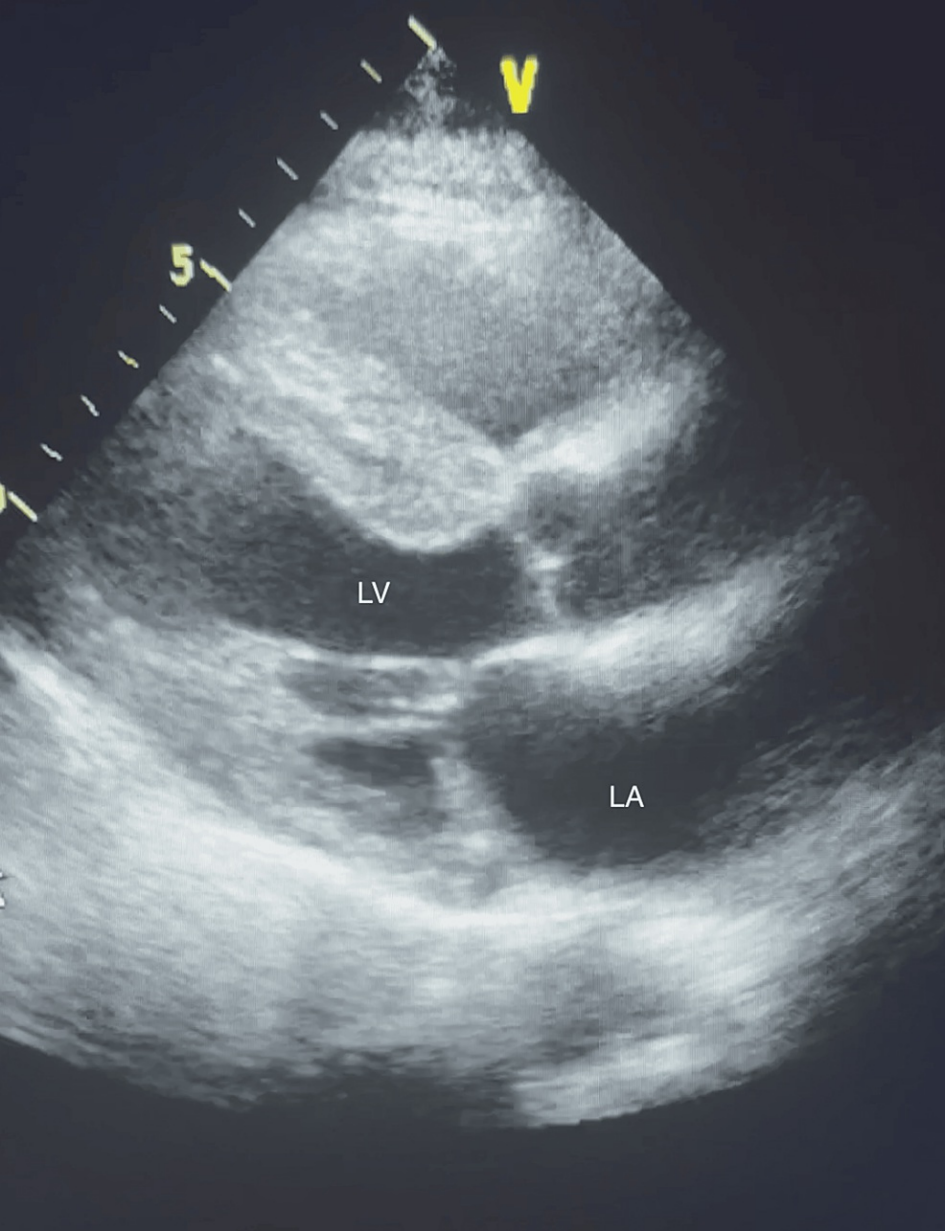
Parasternal long-axis view on TTE 11 days prior to presentation revealing left ventricular hypertrophy LA: left atrium; LV: left ventricle; TTE: transthoracic echocardiogram
